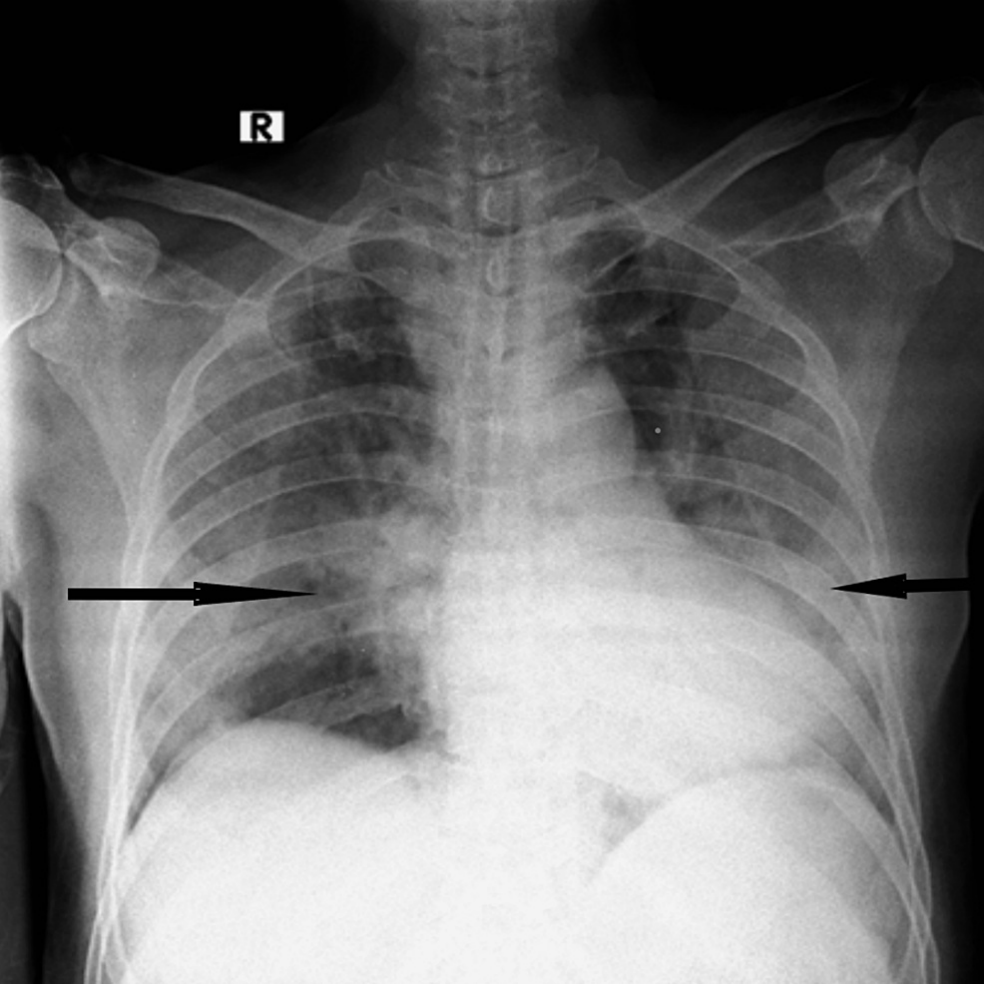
Case 2 Fifty years old COVID-19-positive patient came with complaints of fever, cough, and breathlessness. Her oxygen saturation was 70% under room air, thus high-flow oxygen inhalation was used. She underwent a portable X-ray, which showed consolidatory changes involving bilateral middle lung zones and left lower zones indicated by black arrows.
